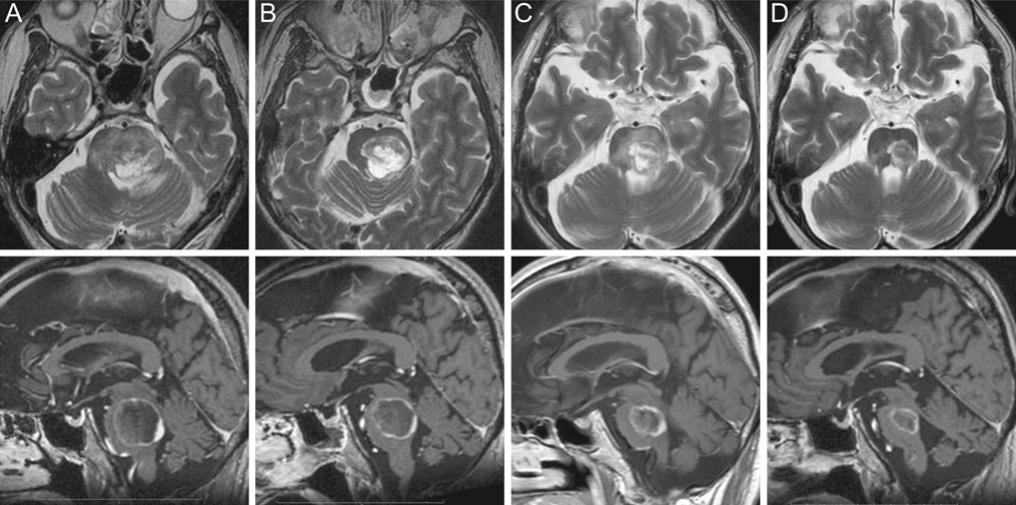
A 72-year-old man with small cell lung cancer presented with reduced conscious level. MR imaging demonstrated a large necrotic metastatic brain tumor in the pons. Due to the severity of neurological symptoms, the risk of WBRT was rated as high. As an alternative treatment option, the patient was allocated to two-session GKS. The first treatment delivered 10 Gy to the 40 % isodose (Fig. 3a). Three weeks later, at the second session, significant tumor volume reduction was observed and 10 Gy to the 40 % isodose was delivered to the tumor margin (Fig. 3b). Follow-up MR imaging after 4 months showed a considerable decrease in tumor size but brain stem perifocal edema (Fig. 3c). The perifocal edema subsided by the 8-month follow-up (Fig. 3d), and the KPS improved from 30 to 70. Although transient neurological deterioration occurred due to delayed radiation injury, the patient could lead an independent life until 2 months before he died from systemic disease progression
